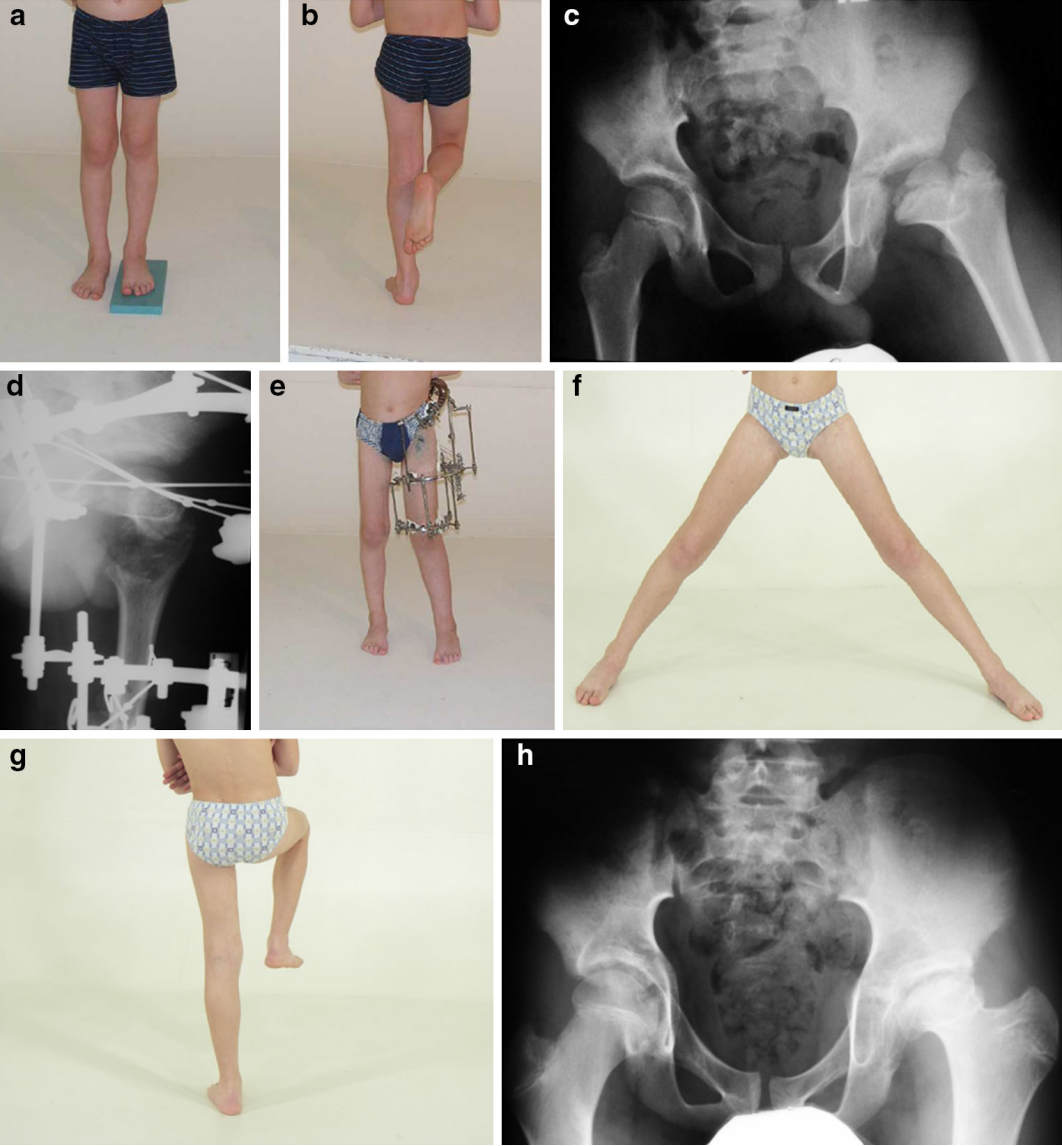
a, b Preoperative left leg shortening and positive Trendelenburg sign in a 6-year-old boy with septic coxitis sequelae, Kalamchi II. c Preoperative AP pelvic radiograph with characteristic left proximal femoral ischemic deformities. d Neck lengthening with regenerate formation. e Patient with apparatus during the consolidation phase. f, g Three years postoperative abduction and negative Trendelenburg sign. h AP radiograph 5 years postoperatively showing remodeling of the left proximal femur
